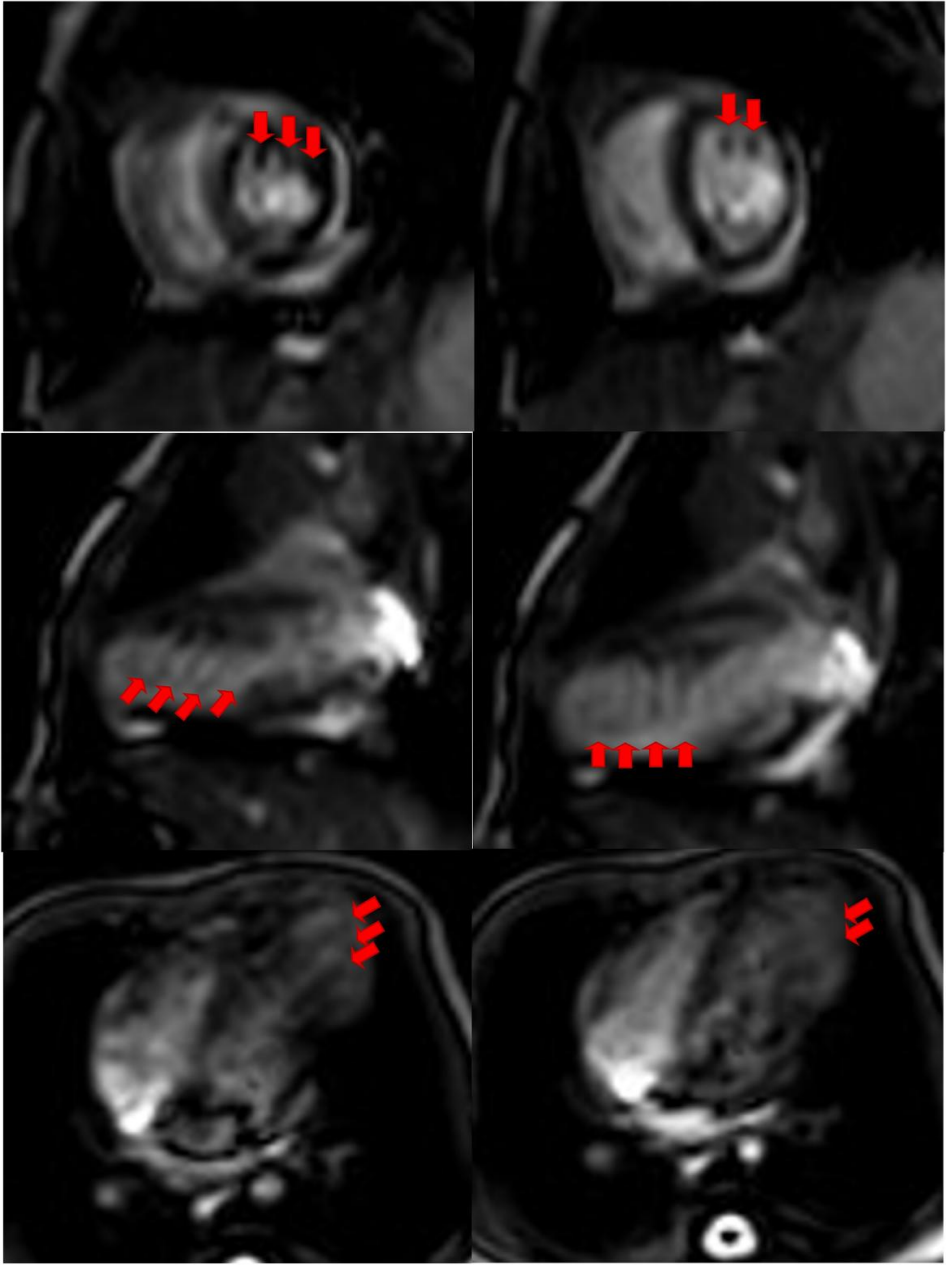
Saw-tooth cardiomyopathy is visible during the systolic phase and relaxation phase of cardiac magnetic resonance imaging. Protrusion of muscular bridges in the left ventricle mimicking saw-tooth-like projections (arrows) can be seen.
